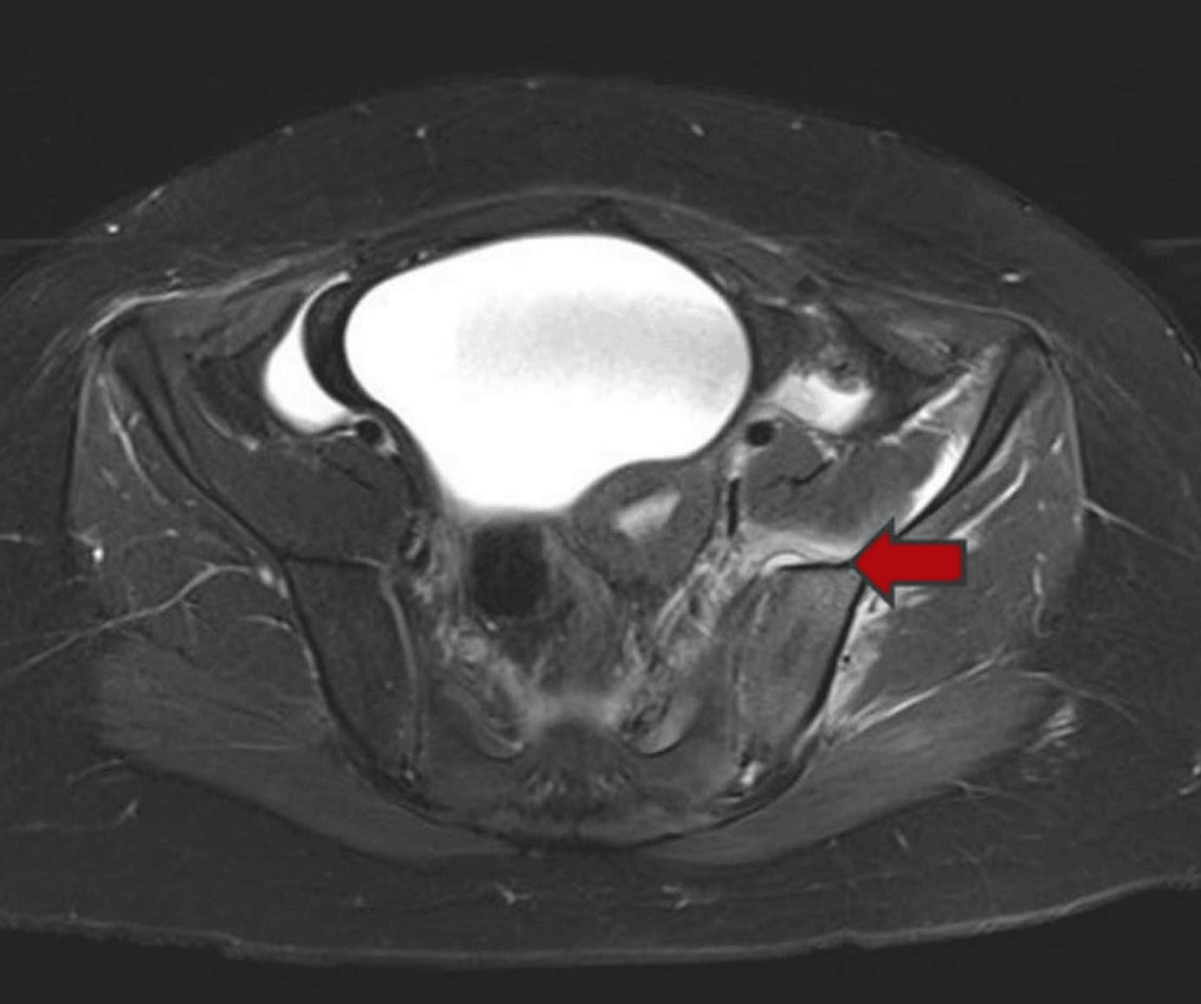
Pelvic MRI (day 3) showing left sacroiliac septic arthritis with adjacent osteomyelitis and abscess
